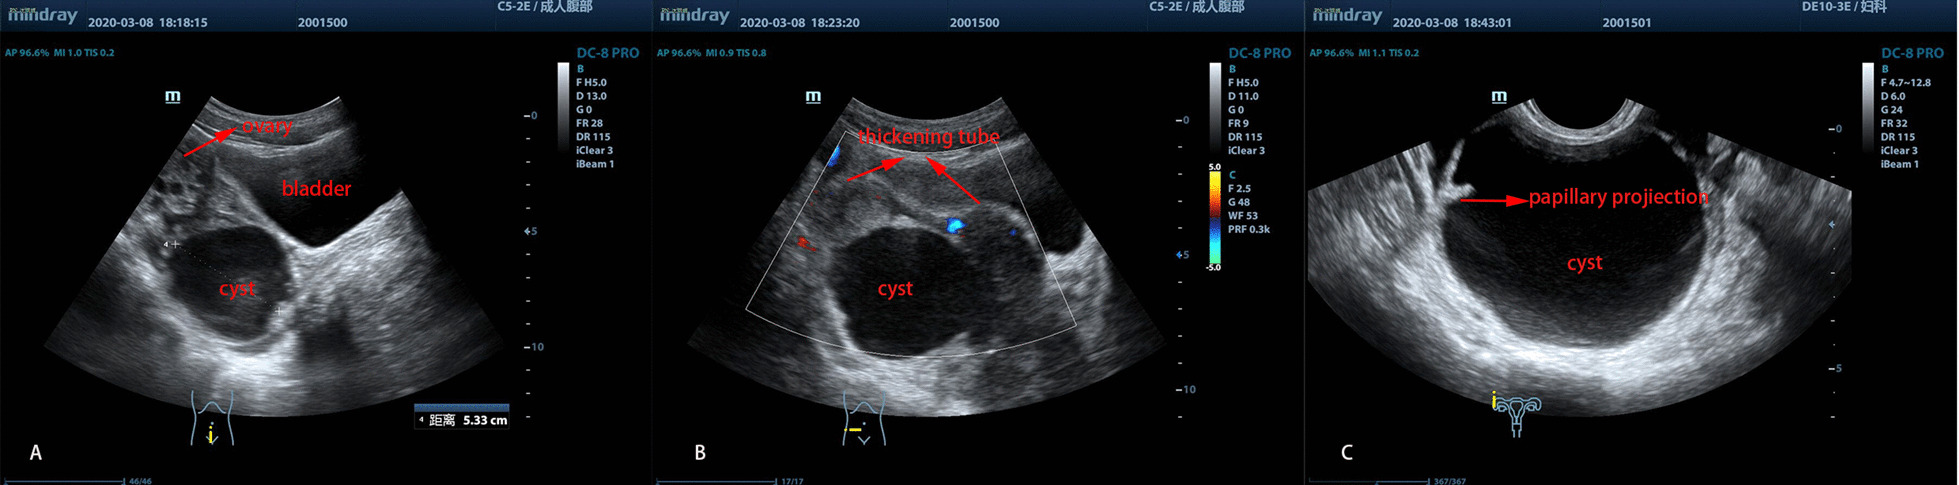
Ultrasonographic identification of a cystic mass in the right lower abdomen A a normal ovary with a cystic mass underneath it. B Thickening tube beside the cyst. C The papillary projection in the cyst wall
